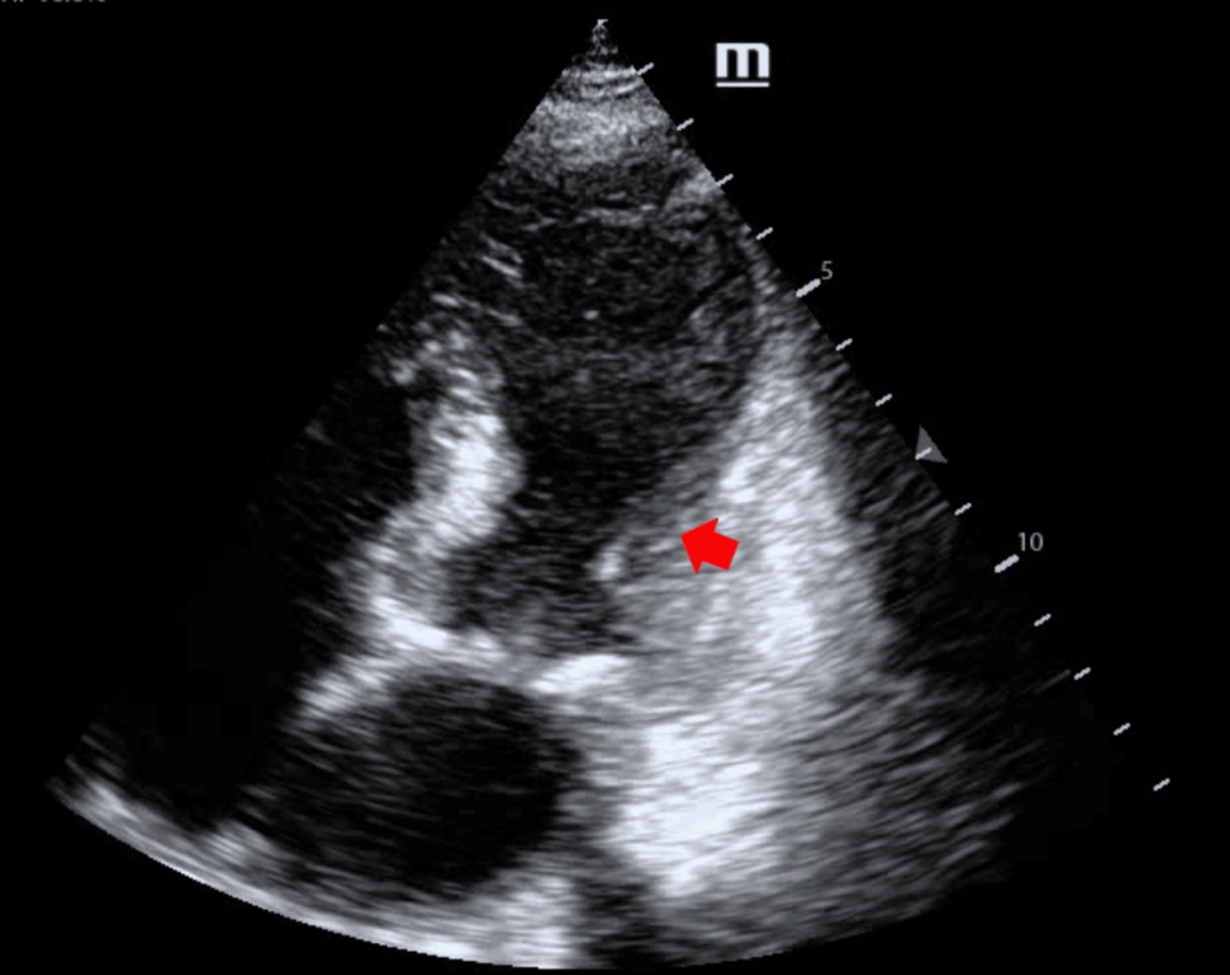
Transthoracic echocardiogram, apical four-chamber view Shows regional abnormalities of left ventricular wall motion, characterized by hypokinesis of the mid-apical segments with preserved basal contraction and hyperdynamic activity, a pattern consistent with stress-induced cardiomyopathy (Takotsubo syndrome) (red arrow).
